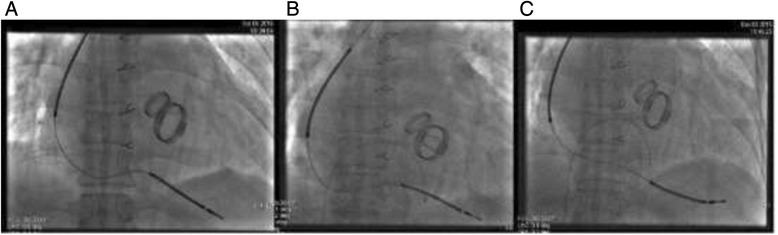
Anteroposterior chest X-ray follow-up of the ICD lead position. a ICD lead position after first implant. b No apparent dislocation of the lead at 9 weeks post- first implant. c Chest X-ray image of lead reposition. ICD, implantable cardioverter-defibrillators
